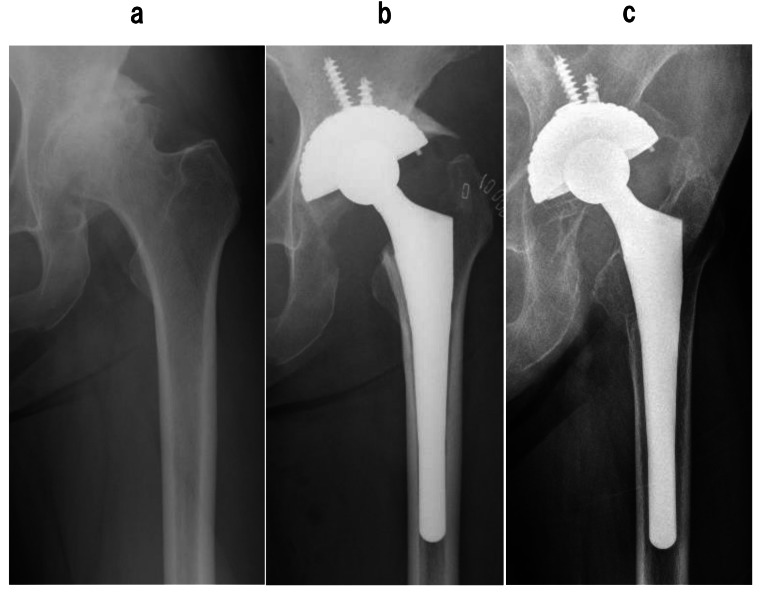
Stress shielding around anatomic fit stem. (a) Preoperative radiographs showed that femur were classified Dorr B. (b) Immediately after primary cementless THA, stem alignment was good position and canal filling was enough. (c) Followed up 21 years after primary operation, this femur was classified grade 3 stress shielding
